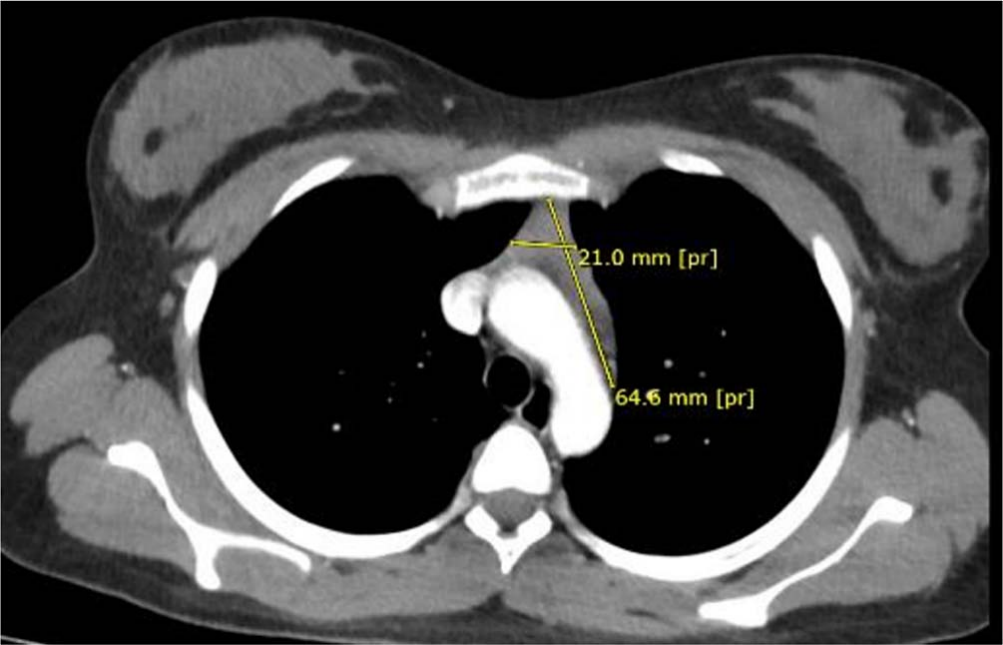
Preoperative thoracic CT showing a 61 × 24 mm homogeneous anterior mediastinal mass.
